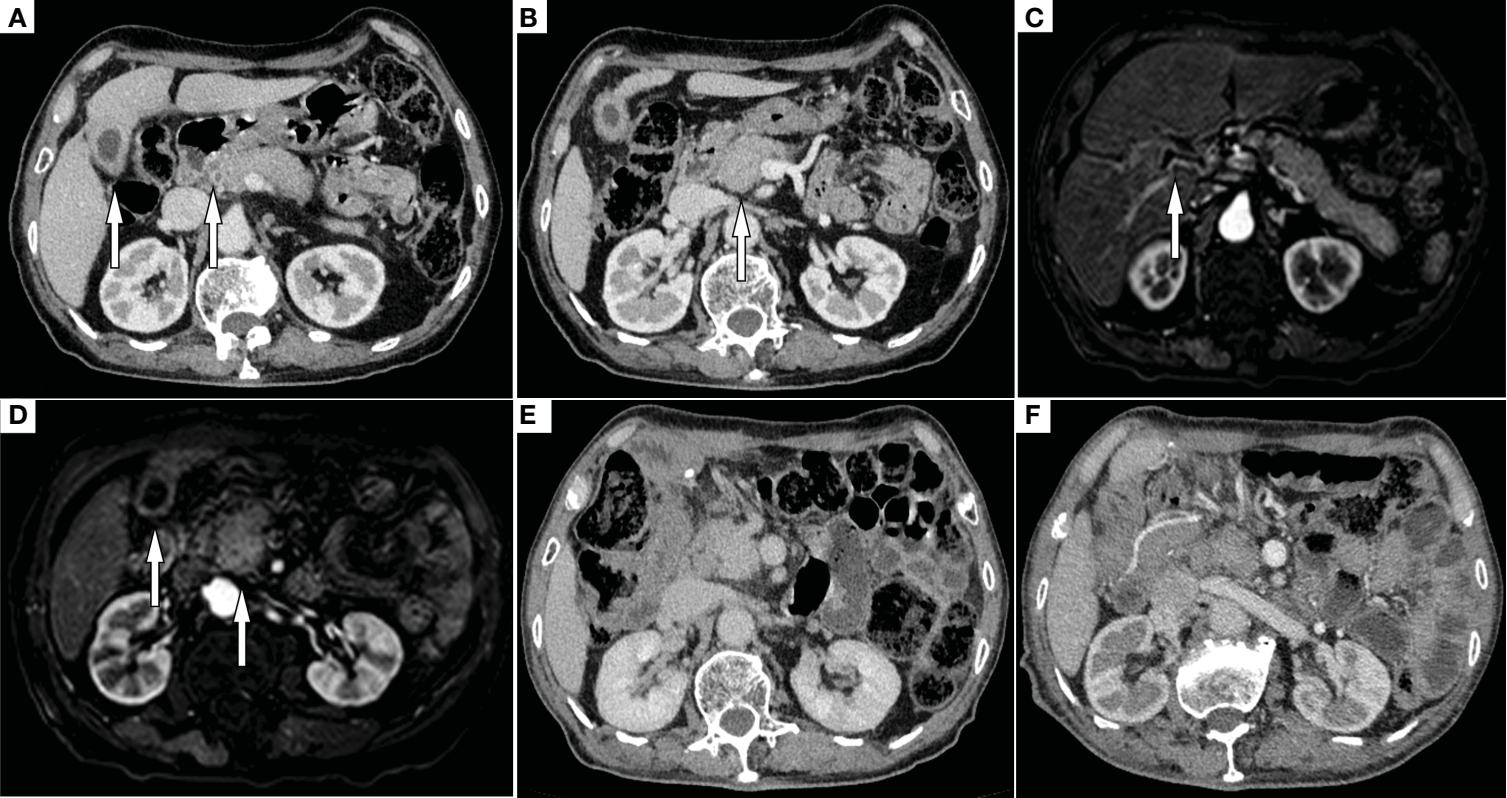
CT (A) Arrows indicate thickened gallbladder wall and thickened common bile duct; (B) Arrows indicate enlarged pancreatic head; MRI (C) Arrows indicate dilated extrahepatic bile ducts; (D) Arrows indicate thickened gallbladder wall and enlarged pancreatic head; (E) Follow up CT at 4 months after surgery; (F) Subsequent CT performed at 9 months post-surgery revealed no significant abnormalities in the pancreas.
